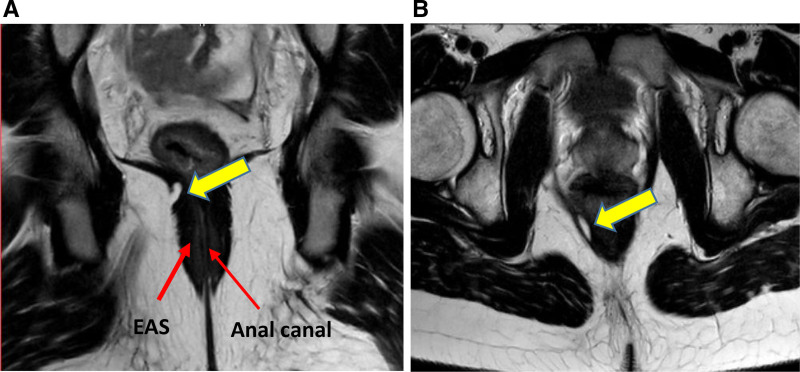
Pelvic MRI showing: (A) T2-coronal oblique and (B) T2-axial views. MRI = magnetic resonance imaging. The yellow arrows show the fat protrusion through the external anal sphincter (EAS) defect reported only at 8 o’clock (lithotomy position). The anal canal in collapsed in the midline (figure 1A) with no abnormality identified in the internal anal sphincter (IAS), (thin wall lining the anal canal).
